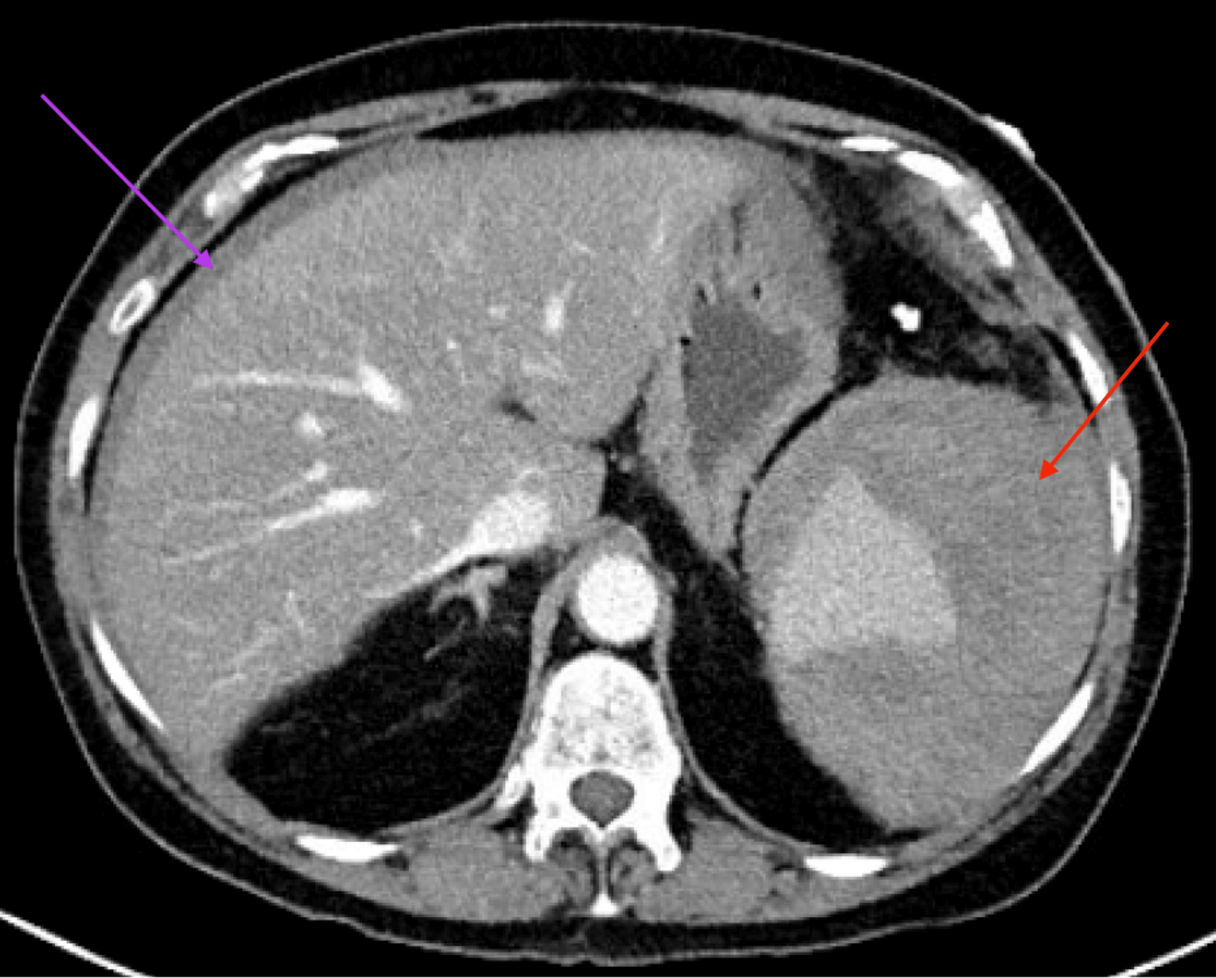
Computed tomography of the abdomen and pelvis Axial view demonstrates the perisplenic hematoma (red arrow) with associated hemoperitoneum (purple arrow).
